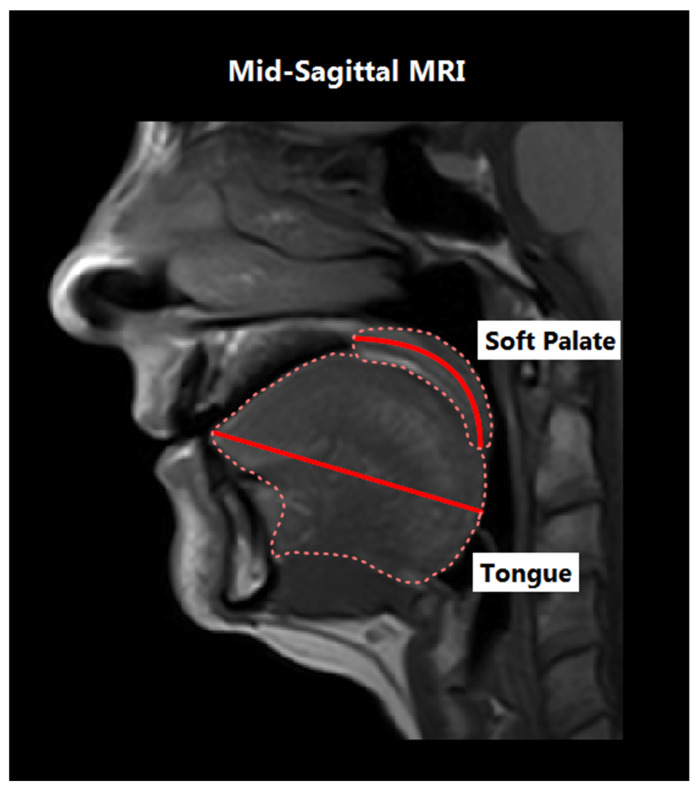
Soft Tissue Segmentation. Axial T1 spin-echo MRI slice demonstrating volumetric segmentation tongue, soft palate, medial pterygoids, parapharyngeal fat pads, lateral pharyngeal walls, and airway. Segmentations were performed using a combination of manual and semi-automated techniques.
